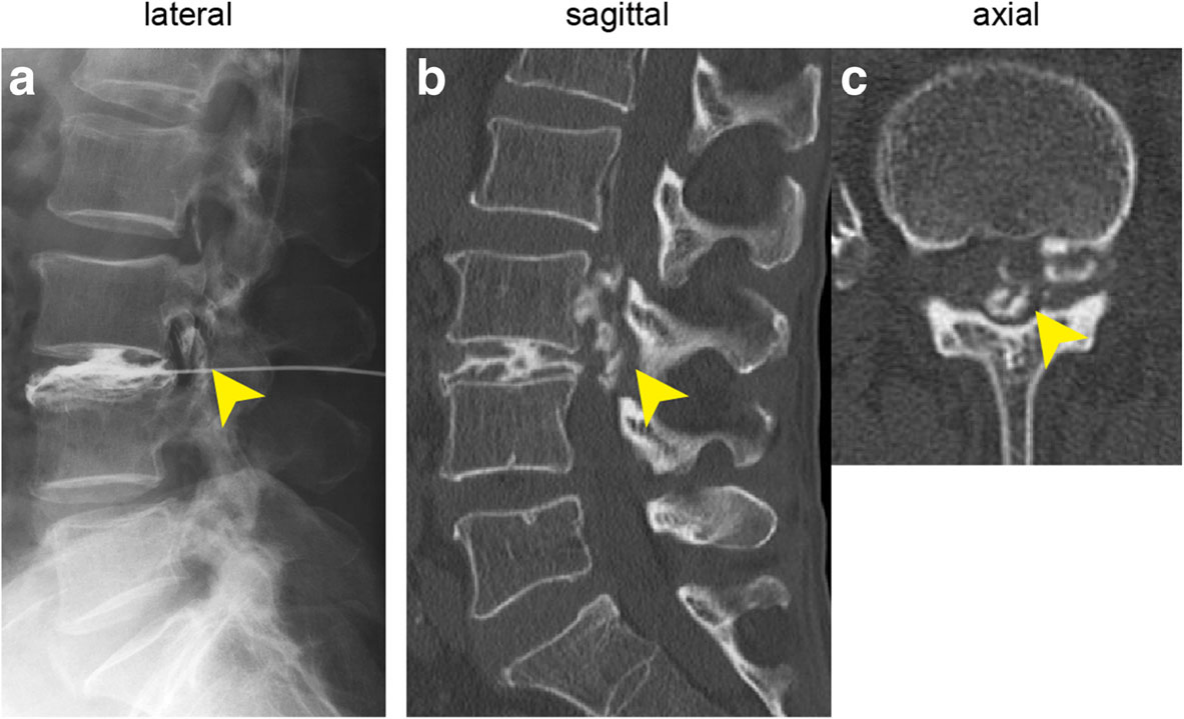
Discography and disco-computed tomography (CT). a Lateral view. (b, c) Sagittal and axial disco-CT view. Discography and disco-CT clearly revealed leakage of the contrast medium into the dorsal canal space from the L3/4 disc level (arrowheads)
